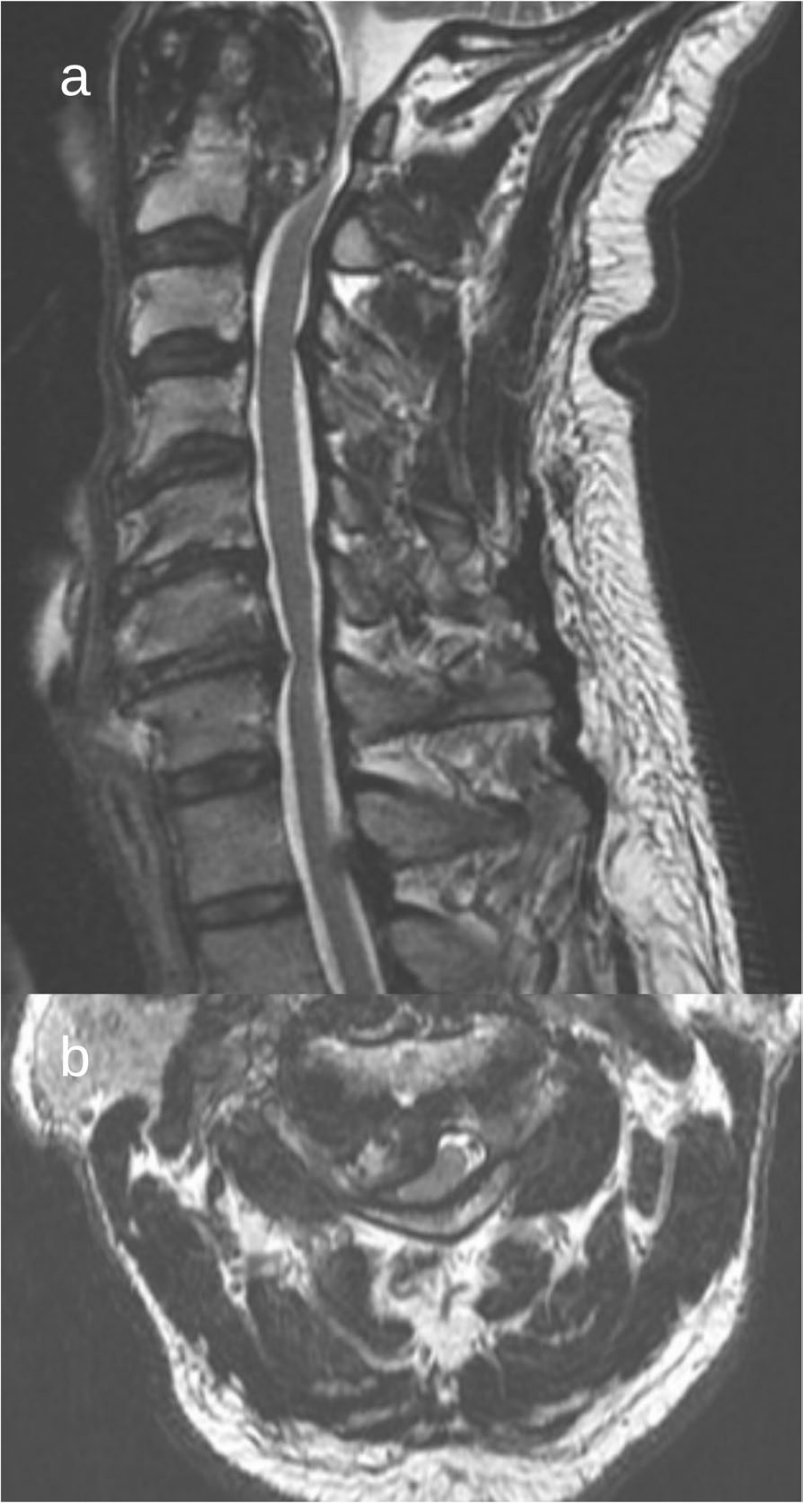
Preoperative MRI T2-weighted imaging: sagittal (a) and axial (b) views suggesting a congenitally narrow cervical spinal canal and hypointense mass causing significant spinal cord compression at C1 and C2
